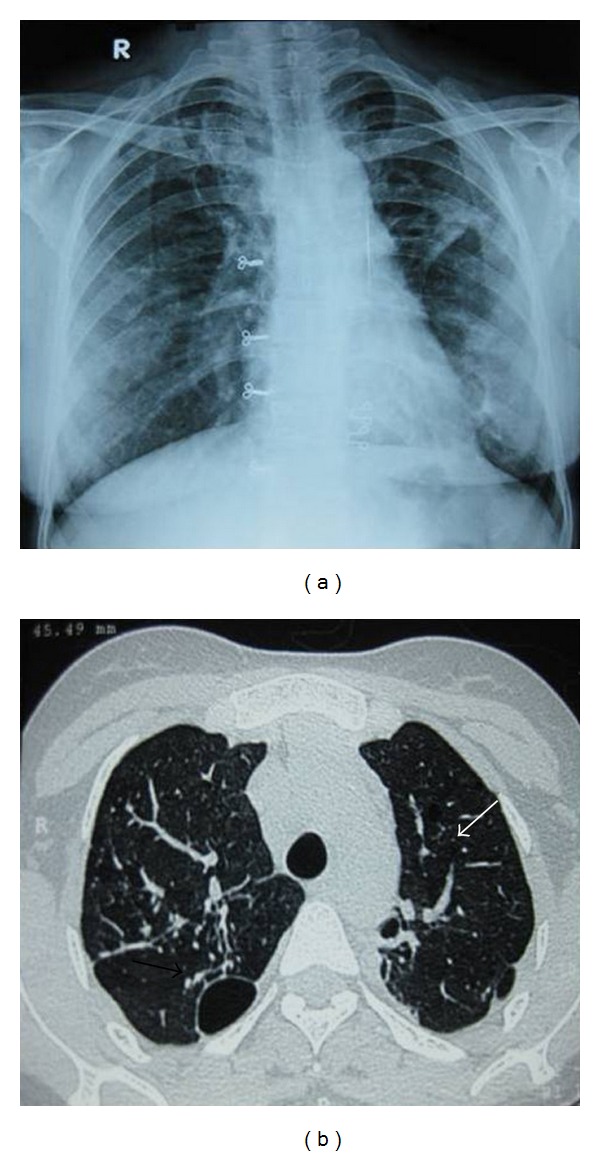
Follow-up (at end of chemotherapy) chest X-ray (a) showing complete resolution of pneumothorax on left side and computed tomography (b) revealing persistence of cystic space in right upper lobe (black arrow) and resolution of left pneumothorax (white arrow).
